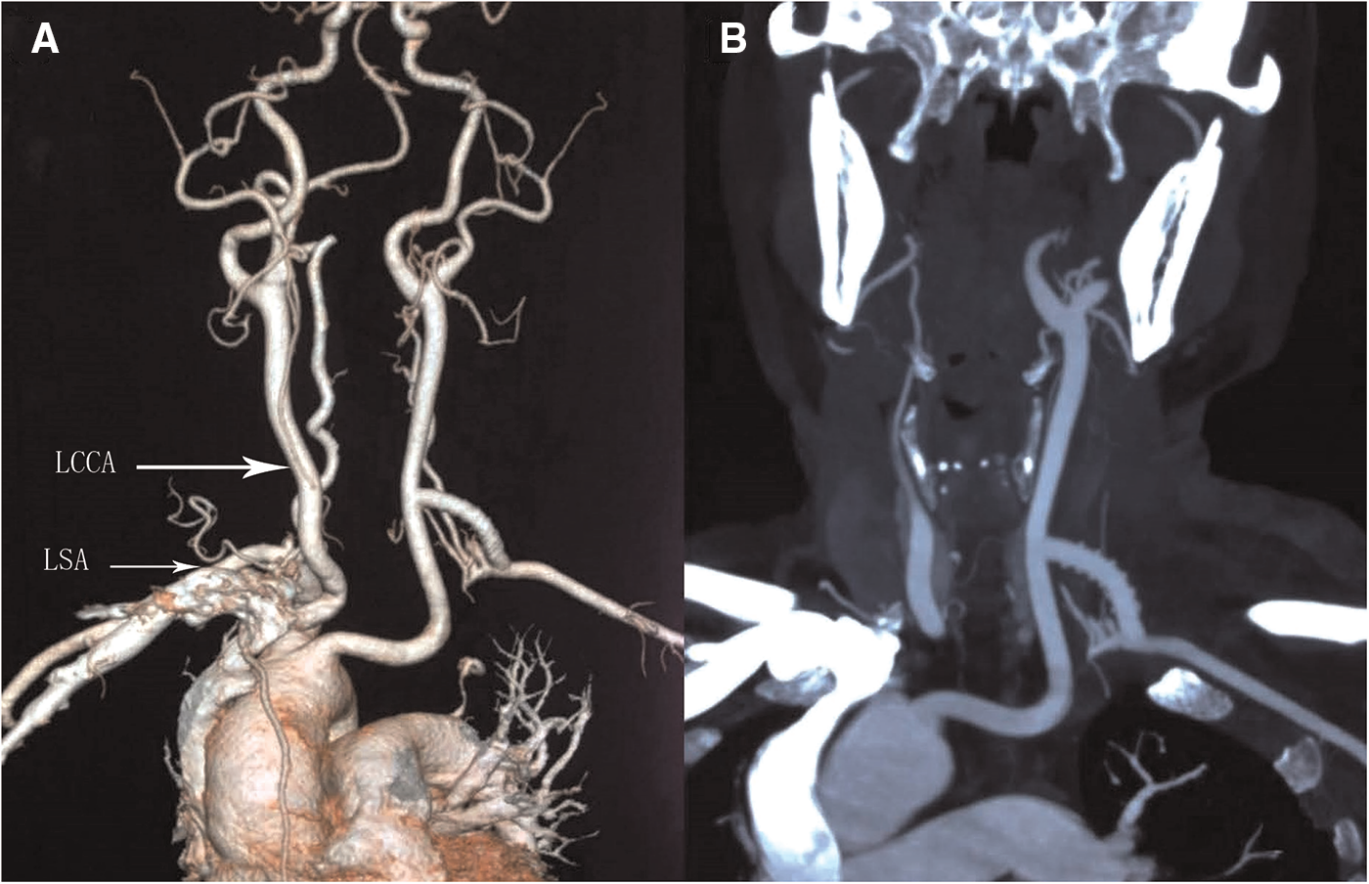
Postoperative CTA showed that good angiography of bypass vessels (A,B). LSA, left subclavian artery; LCCA, left common carotid artery.
